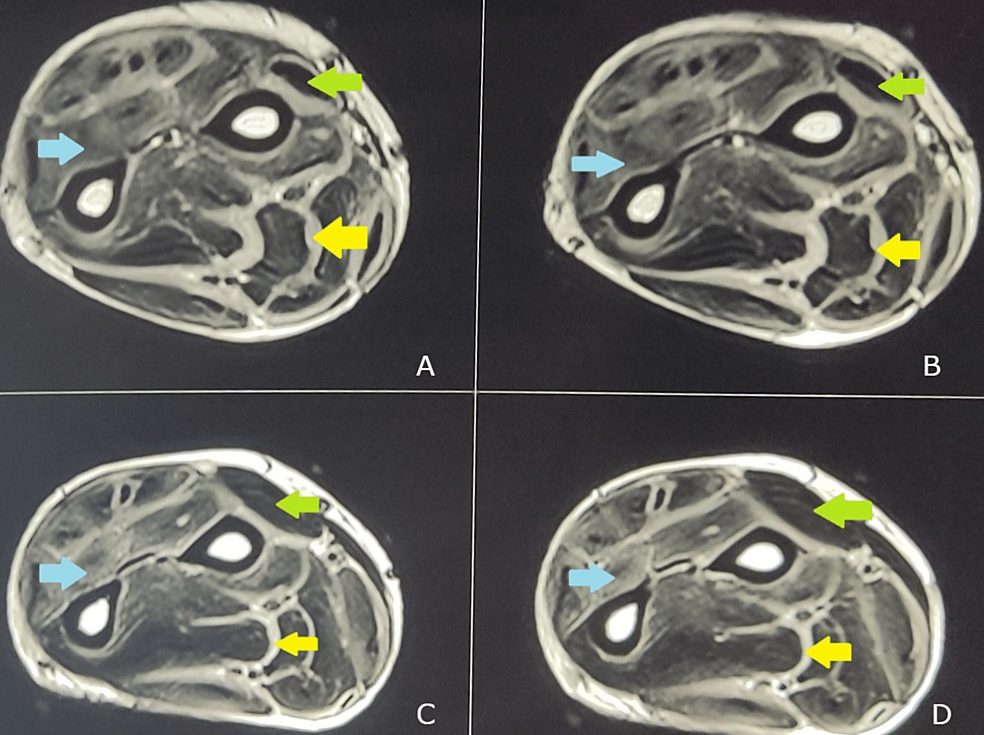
Axial magnetic resonance imaging scan sections showing the presence of an inflammatory pathology The axial sections show the obliteration of intermuscular fascial planes with thickening (yellow arrow). Due to the presence of an inflammatory pathology, the muscles appear whiter than normal (blue arrow). The relatively spared muscles that appear darker have been marked for comparison (green arrow).
